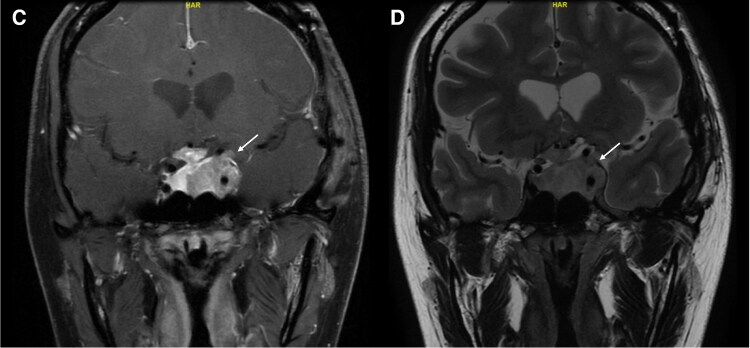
(C) Coronal section of T1-weighted postgadolinium MRI images of the brain postoperative. (D) Coronal section of T2-weighted MRI images of the brain postoperative. Abbreviation: MRI, magnetic resonance imaging.
